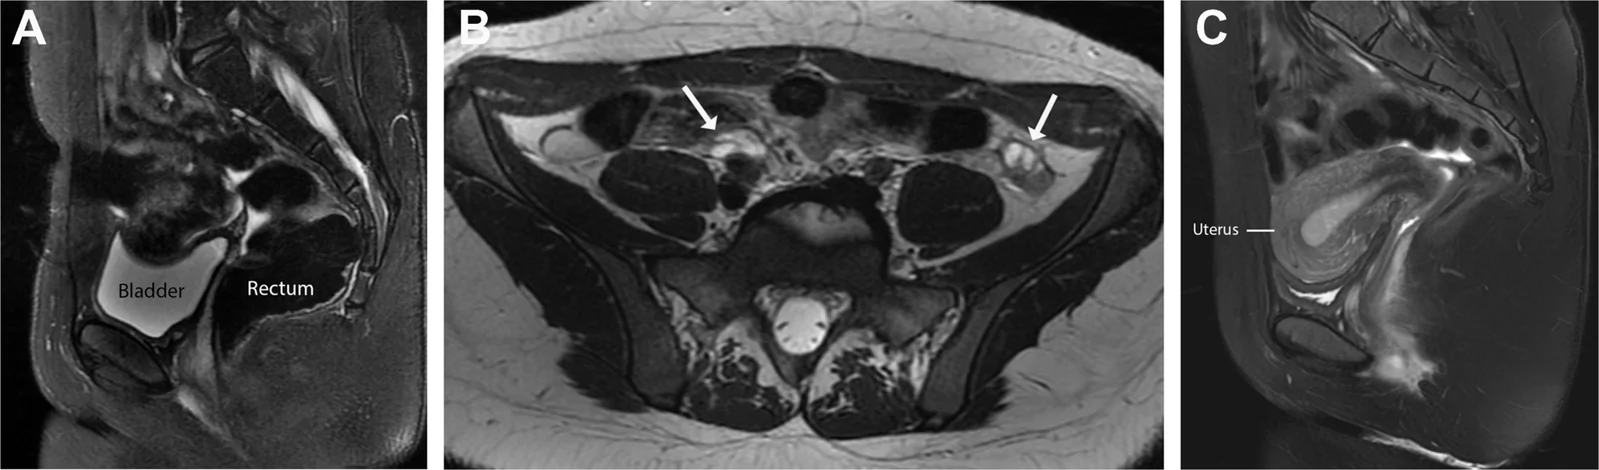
A 14-year-old female with primary amenorrhea and Müllerian agenesis on MRI. a Sagittal T2-weighted fat-suppressed image of the midline pelvis shows absence of the uterus posterior to the bladder. b Axial T2-weighted image shows presence of bilateral ovaries (*arrows*). c In comparison, a sagittal T2-weighted image through the midline pelvis of a normal 17-year-old female shows expected position of an anteverted uterus
